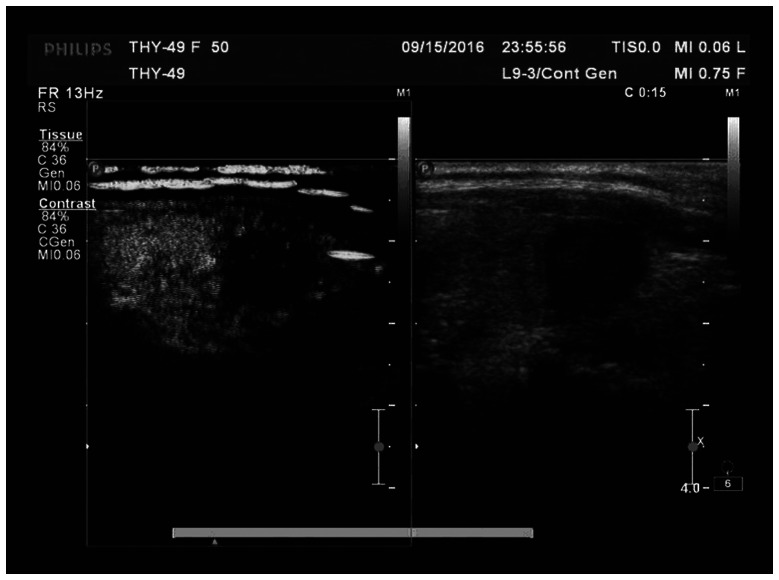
Weak enhancement by CEUS (pathologically diagnosed as PTC). CEUS, contrast-enhanced ultrasonography; PTC, papillary thyroid carcinoma.
